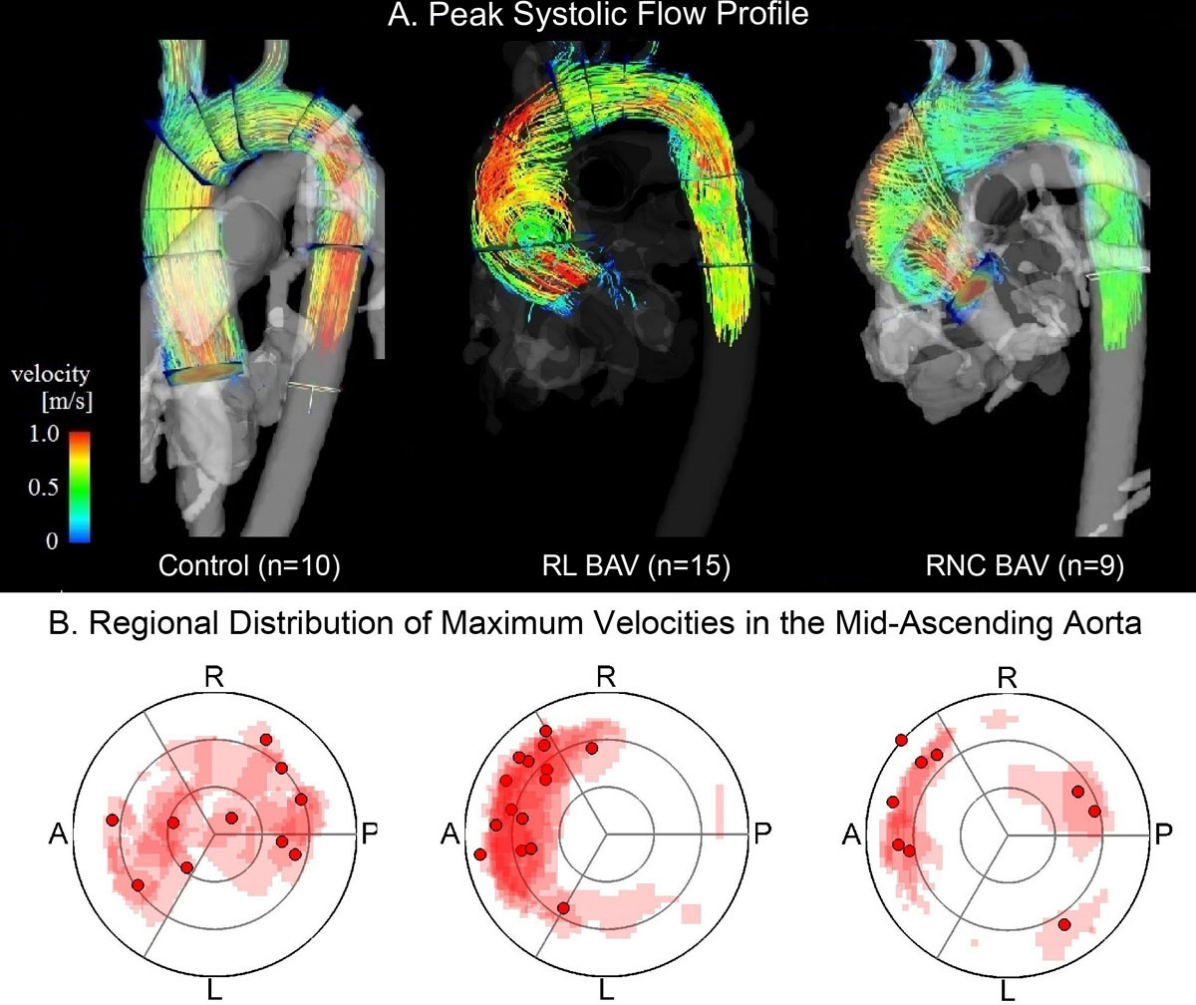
(A.) 4D flow MRI visualization of peak systolic flow using 3D pathlines (color-coded for velocity gradient) demonstrated asymmetric helical flow in RL- and RNC- BAV patients compared to more uniformly distributed flow in trileaflet controls. (B.) Locations of the top 15% of systolic velocities (mapped across a 2D analysis plane in the mid-ascending aorta) were centrally distributed in controls and concentrated towards the outer aortic wall in BAV patients. RNC-BAV subjects showed more variation in distribution compared to RL-BAV, whose flow profile was more consistently directed towards the right-anterior wall.
